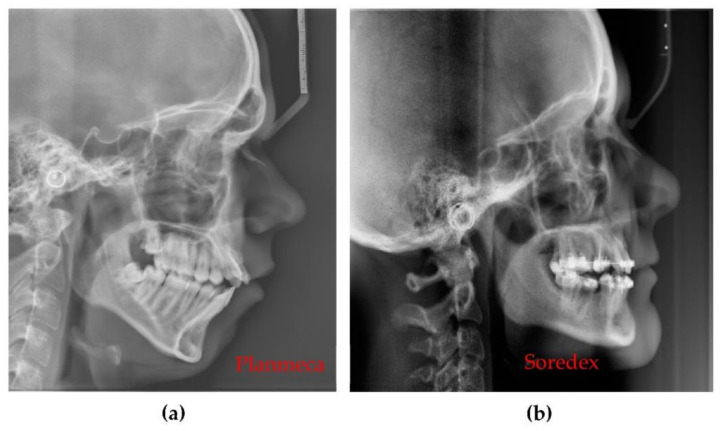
Cephalometric radiographs performed with (a) the Planmeca unit and with (b) the Soredex unit.
